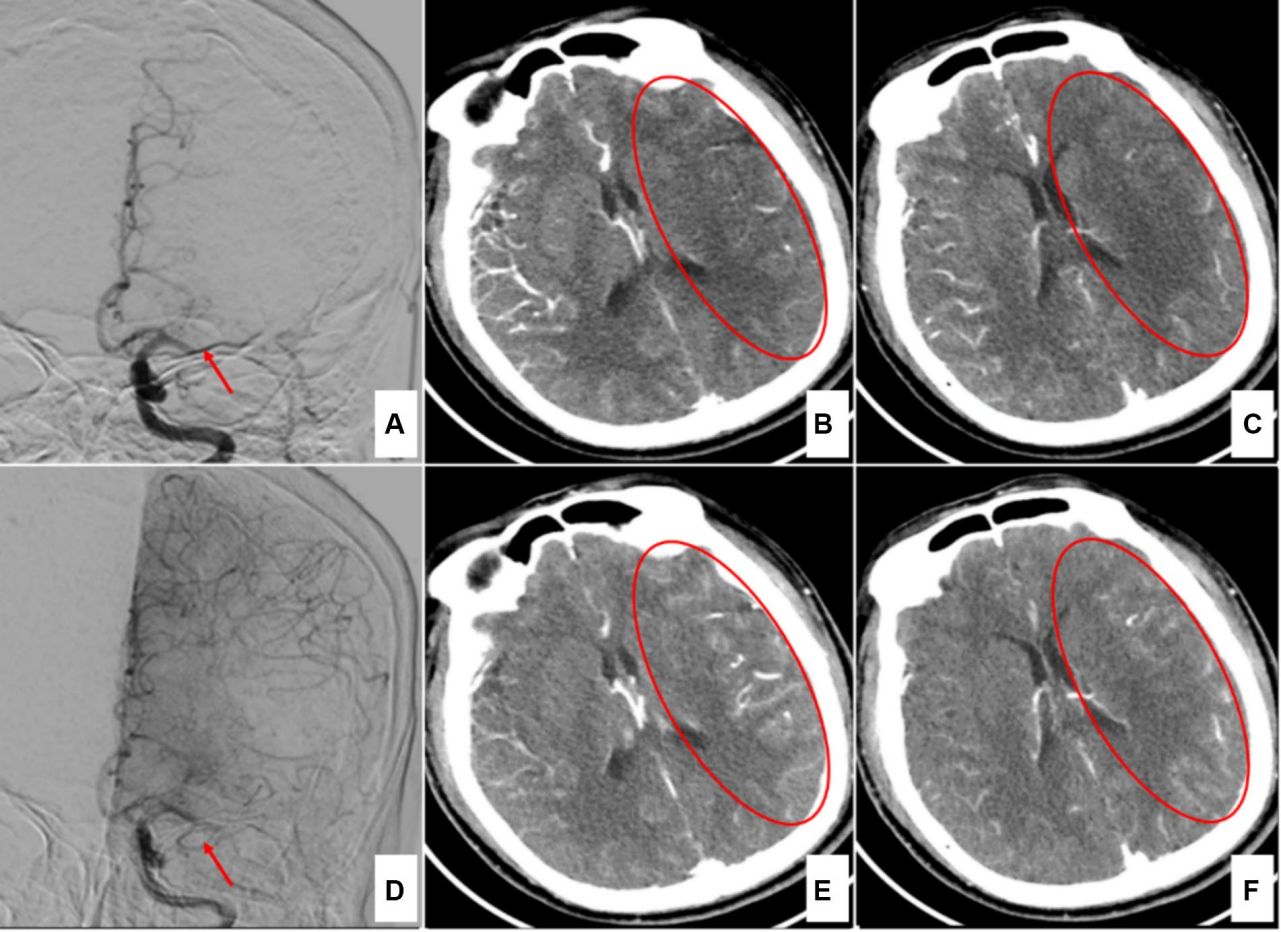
A case of left middle cerebral artery occlusion 4 h after onset. (A) Digital subtraction angiography (DSA) in the early arterial phase indicates left middle cerebral artery occlusion (red arrow). (B,C) Arterial-phase CTA source images show the ischemic region in the left temporal lobe (red circles). (D) DSA in the late arterial phase shows compensatory vessels of the anterior cerebral artery (red arrow). (E,F) Delayed-phase CTA source images indicate that the ischemic region has been filled by the compensatory vessels (red circles).
